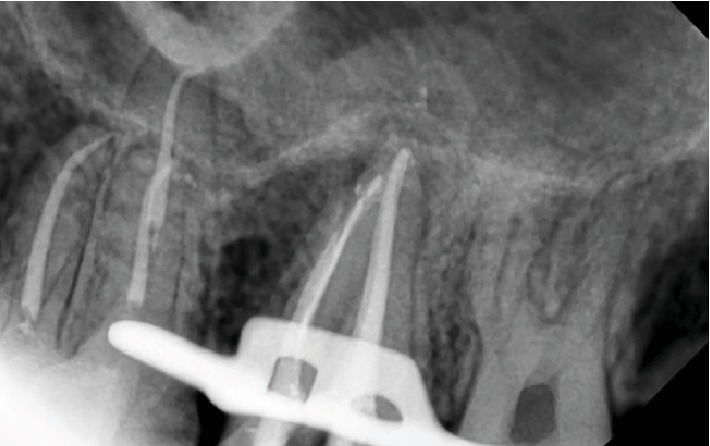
Periapical due to upper 7 obturation.
